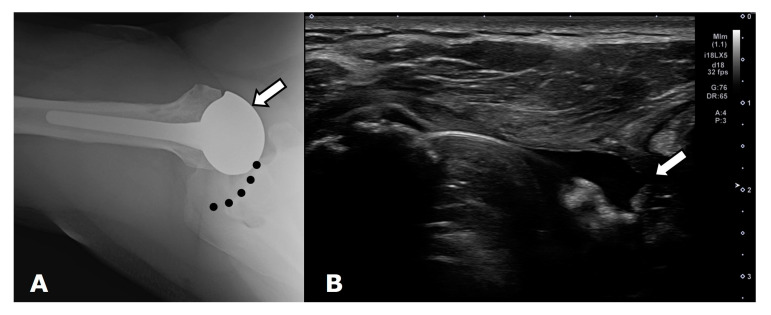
(A). Axillary radiograph shows anterior subluxation of the humeral head (arrow) component of the HA from the glenoid (dotted line) in a patient with subscapularis insufficiency. (B). Long-axis US image (lateral image left, medial image right) in a different patient with an ATSA shows a full thickness subscapularis tendon tear with a retracted tendon stump (arrow).
